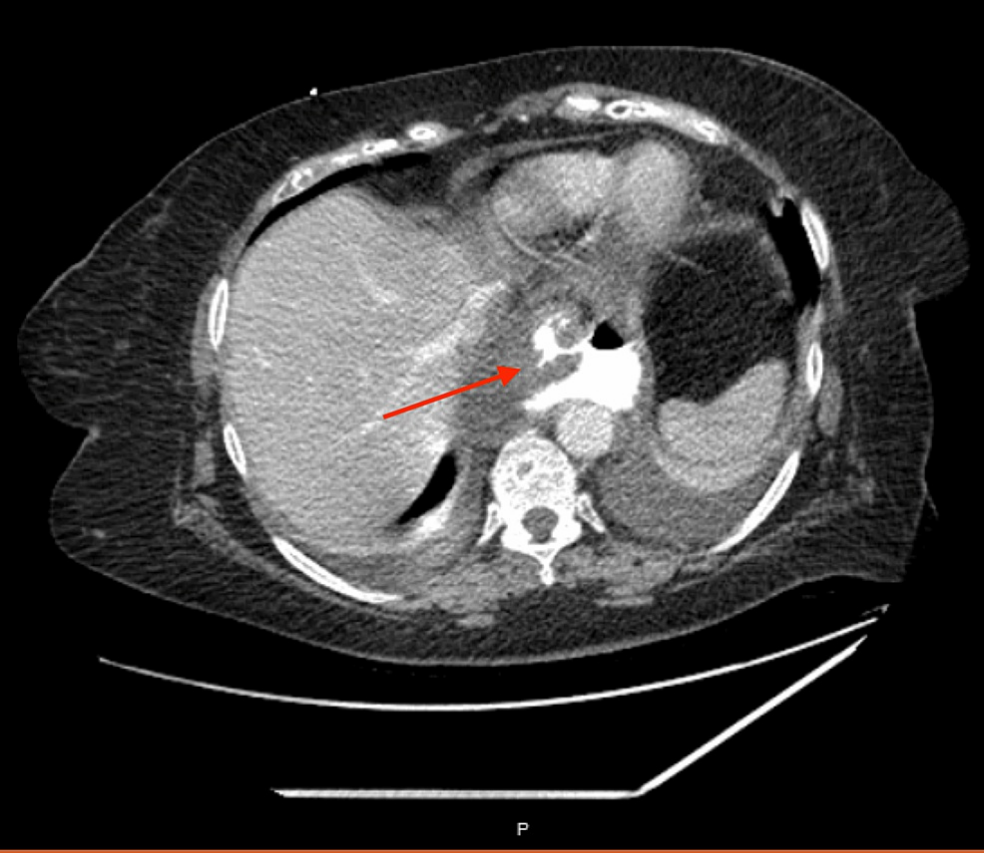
Initial CT scan revealing recurrence of retro-cardiac hernia and extravasation of oral contrast Arrow points towards the retrocardiac hernia and extravasation of oral contrast
